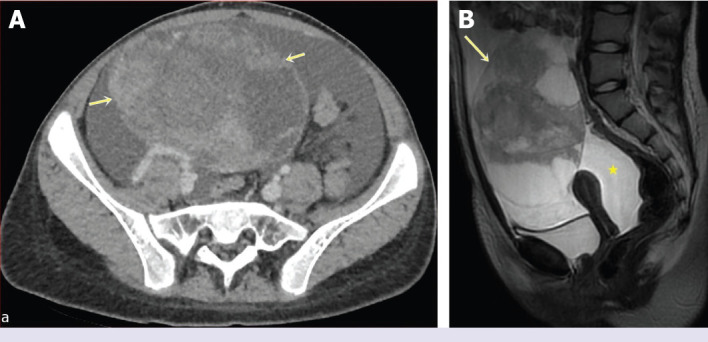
(A) Contrasted computed tomography shows the heterogeneous mass (arrow) originating from the right ovary. (B) Sagittal T2- magnetic resonance image, irregular soft tissue components show significant contrast increase (arrow), accompanying diffuse intraperitoneal free fluid is also seen (star), no abnormality in uterus.
